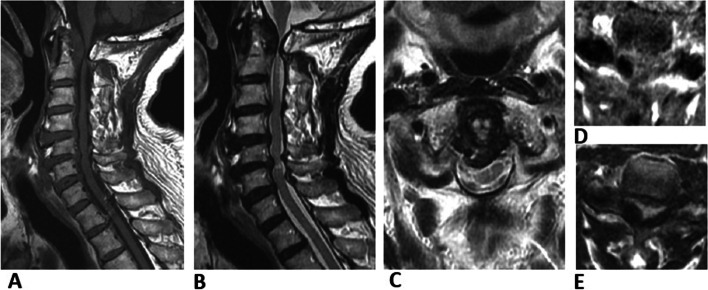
Magnetic resonance imaging of case 15. Nodular masses with low-signal intensity in both T1-weighted imaging (A and D) and T2-weighted imaging (B and E) are seen at the interlaminar spce of C5–6 and C6–7. Magnetic resonance imaging demonstrated a retro-odontoid mass severely compressing the spinal cord (C)
